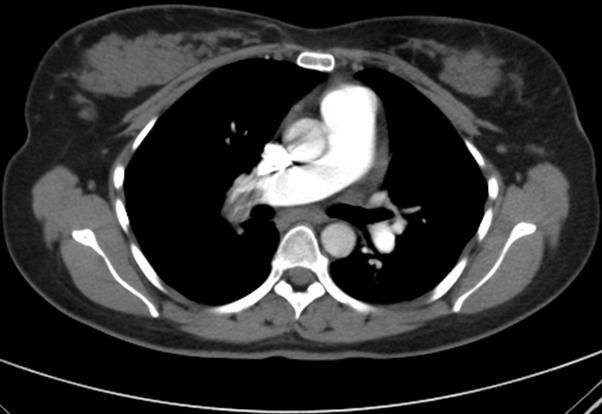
CT-Scan chest with contrast showing bilateral sub-massive pulmonary emboli
